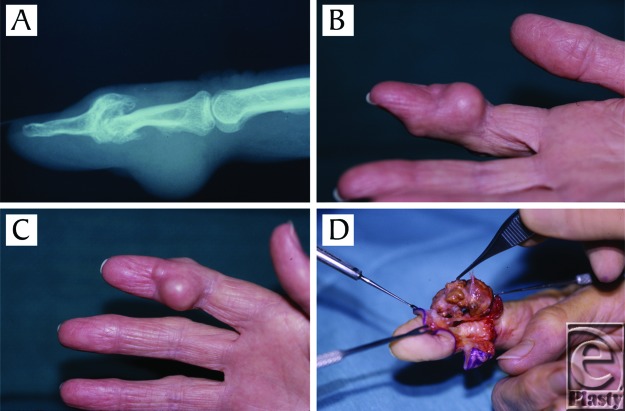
A 64-year-old woman with giant cell tumor of the tendon sheath (GCTTS) on the palmar aspect of the middle phalanx of the right index finger. (*a*) X-ray showing bony erosion. (*b*)-(*c*) Lesion upon presentation. (*d*) Intraoperative view showing typical multilobulated appearance of GCTTS.
